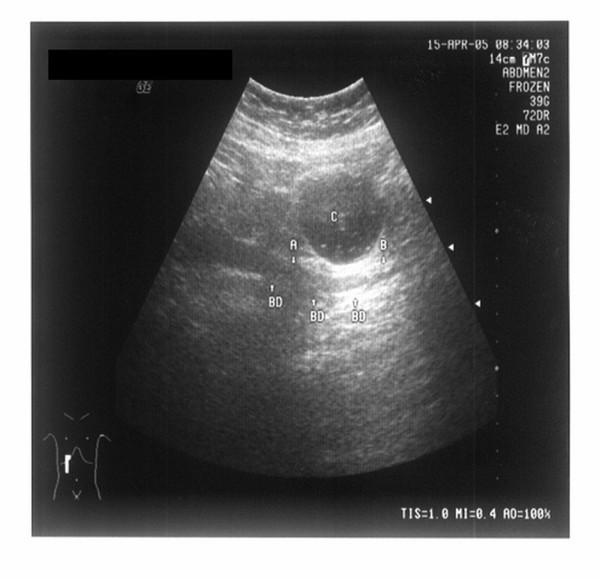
The whole extrahepatic bile duct seen under transabdominal ultrasound after normal saline retention enema. The whole compensatory dilated extrahepatic bile duct (indicated by BD and the arrows) is visible behind the transverse colon (indicated by C). Indicated by A and the arrows is the upper bound of the overlapping between the extrahepatic bile duct and transverse colon, and indicated by B and the arrows is the lower bound of the overlapping. This patient was checked after cholecystectomy, and no calculus or cancer was found, which is not included in the cases studied in this article. It is just taken as an example to describe the effect of visualizing the extrahepatic bile duct after the enema.
